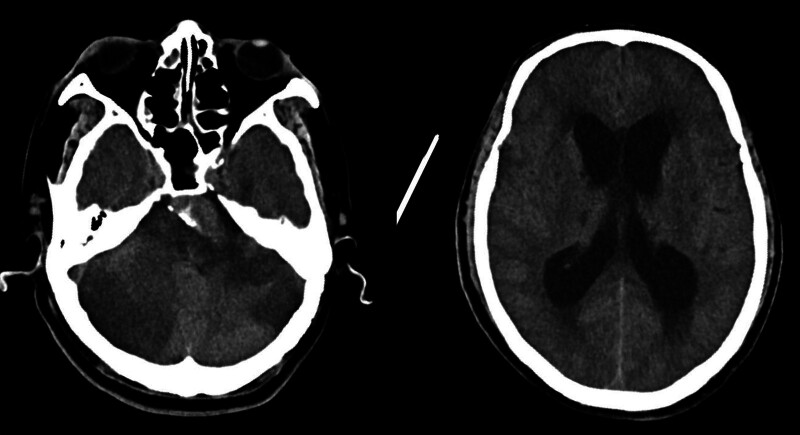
Cranial CT on day 4 revealing the worsening of infarctions in the brainstem and cerebellar hemispheres, with obstructive hydrocephalus. CT = computed tomography.
